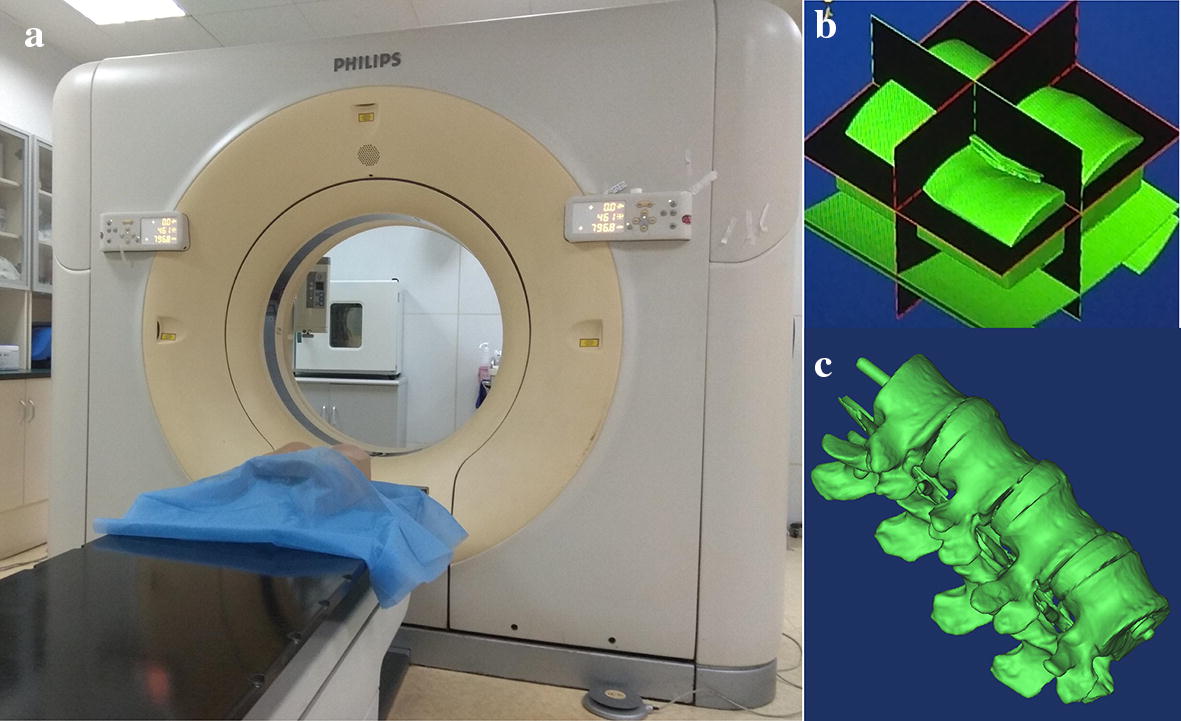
a Spinal model was scanned by CT; b 3D reconstruction of spinal puncture model; c 3D reconstruction model of the spine after removing the skin and flesh
